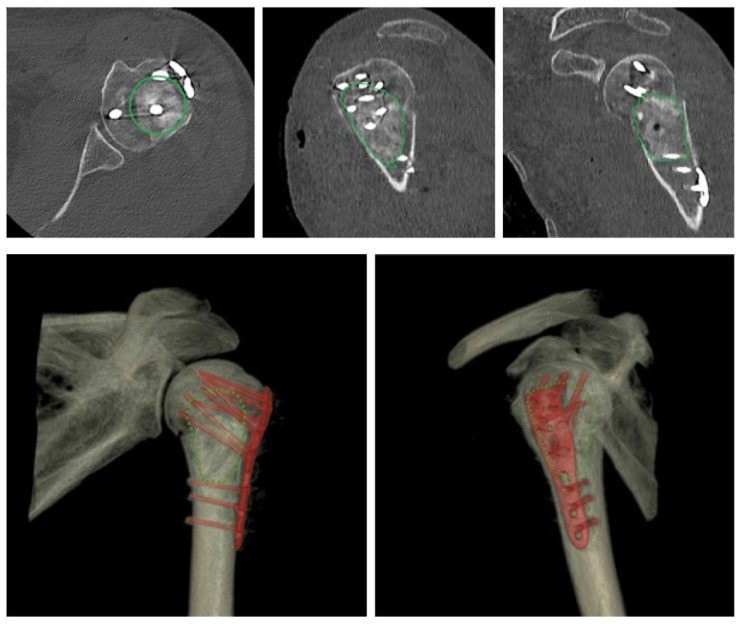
CT imaging of a three-part proximal humerus fracture after surgical treatment using a PHILOS plate with the use of a bone allograft. Imaging in standard planes and 3D reconstruction showing the bone allograft.
